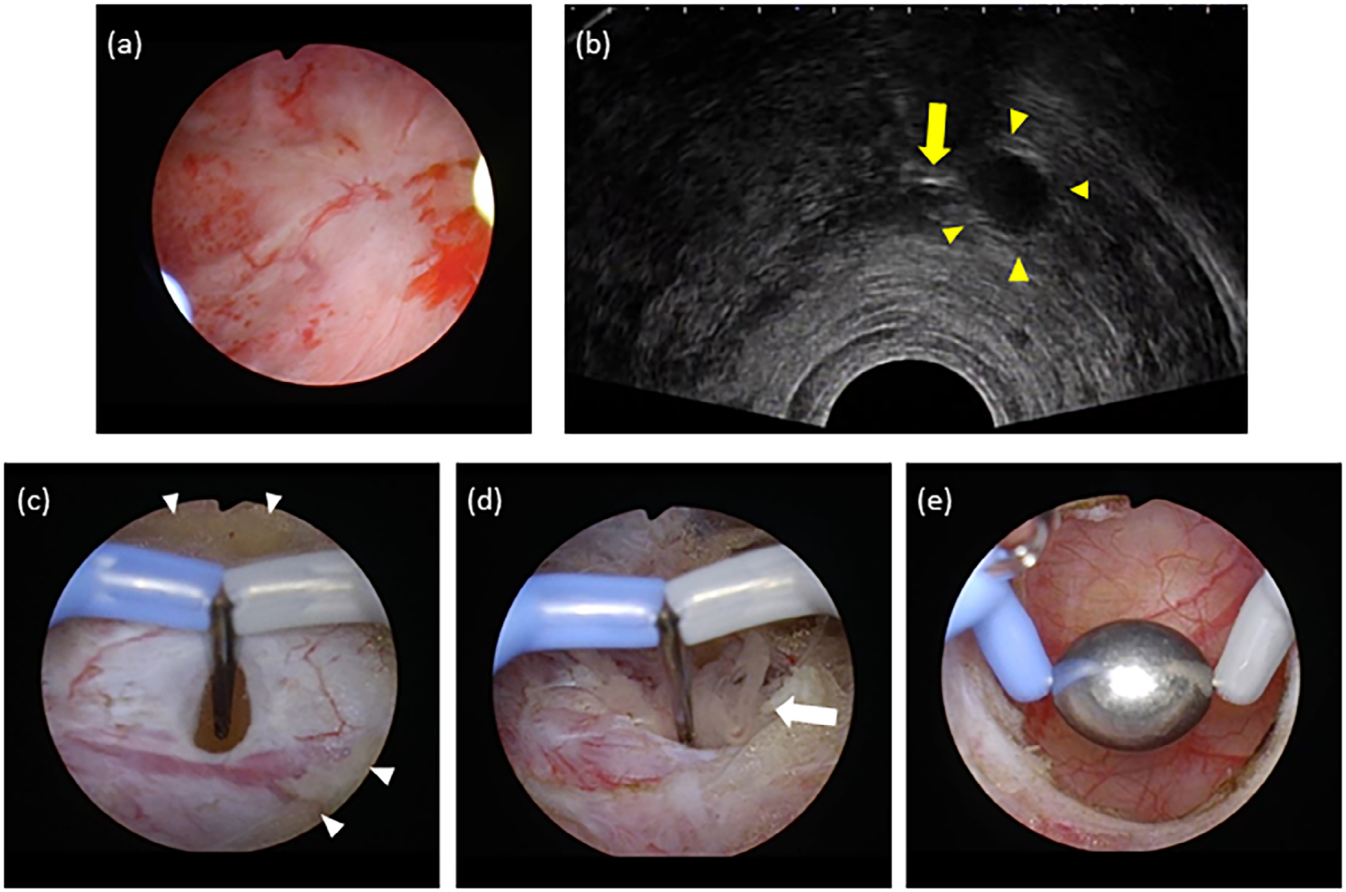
(a) Observation of the bladder lumen shows a flat mucosa behind which the fulgurated diverticulum is located. (b) Transrectal ultrasound findings showing the isolated diverticulum (yellow arrowheads) and the electrode tip (yellow arrow), (c) Incision of the wall of the isolated diverticulum after resection of the fulgurated mucosa. Fat tissues between the diverticulum and the bladder lumen are seen around the diverticulum (white arrowheads). (d) Papillary tumor protruding from the opened diverticulum (white arrow). (e) Lumen of the diverticulum after tumor resection and before fulguration with the roller electrode.
